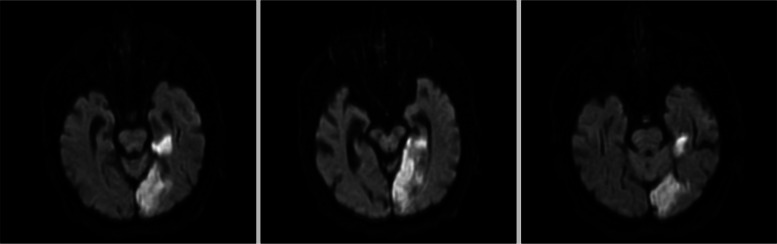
Restricted diffusion in the left occipital lobe on DWI MRI
